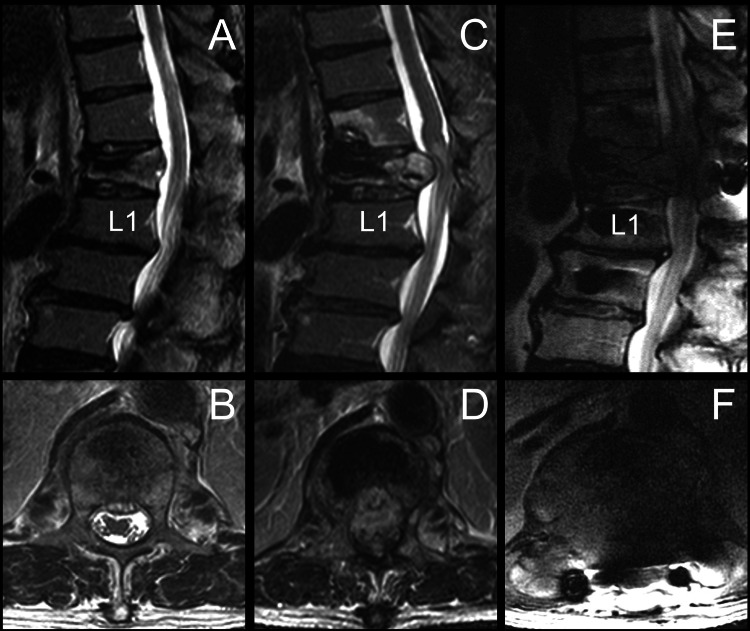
MRI series depicting a T12 compression fracture The MRI series depicting a T12 compression fracture is presented as follows: The initial T2-weighted images ((A) sagittal view; (B) axial view) reveal a T12 compression fracture with heterogeneous signals and no significant extradural compression. Six months following T12 vertebroplasty, the sagittal (C) and axial (D) images show a further reduction in vertebral height and anterior bulging, leading to extradural compression. The patient subsequently underwent a T12 laminectomy with fixation from T10 to L2. However, three months after this secondary surgery, the patient experienced a rapid neurological decline. Follow-up MR images in sagittal (E) and axial (F) views demonstrate progressive extradural compression of the spinal cord. MRI: magnetic resonance imaging
